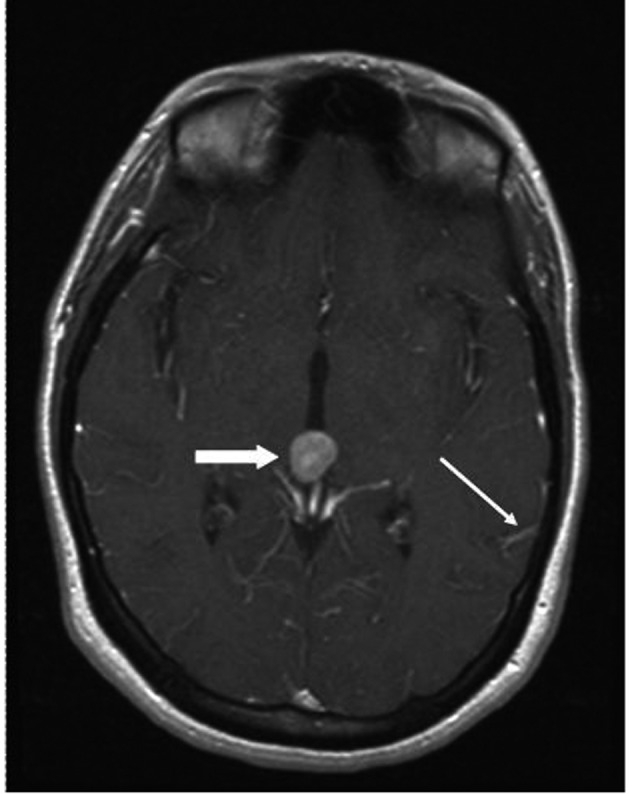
MRI brain (transversal) reveals obstructive hydrocephalus caused by an enhancing pineal gland mass (large arrow) and leptomeningeal carcinomatosis (LC) (small arrow).
